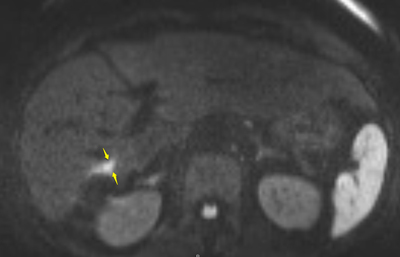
Diffusion-weighted MRI at the same level than Figure 1 showed diffusion restriction in the nodule and the bile duct, characterized by a marked high signal intensity (b-factor value = 800 s/mm2).
